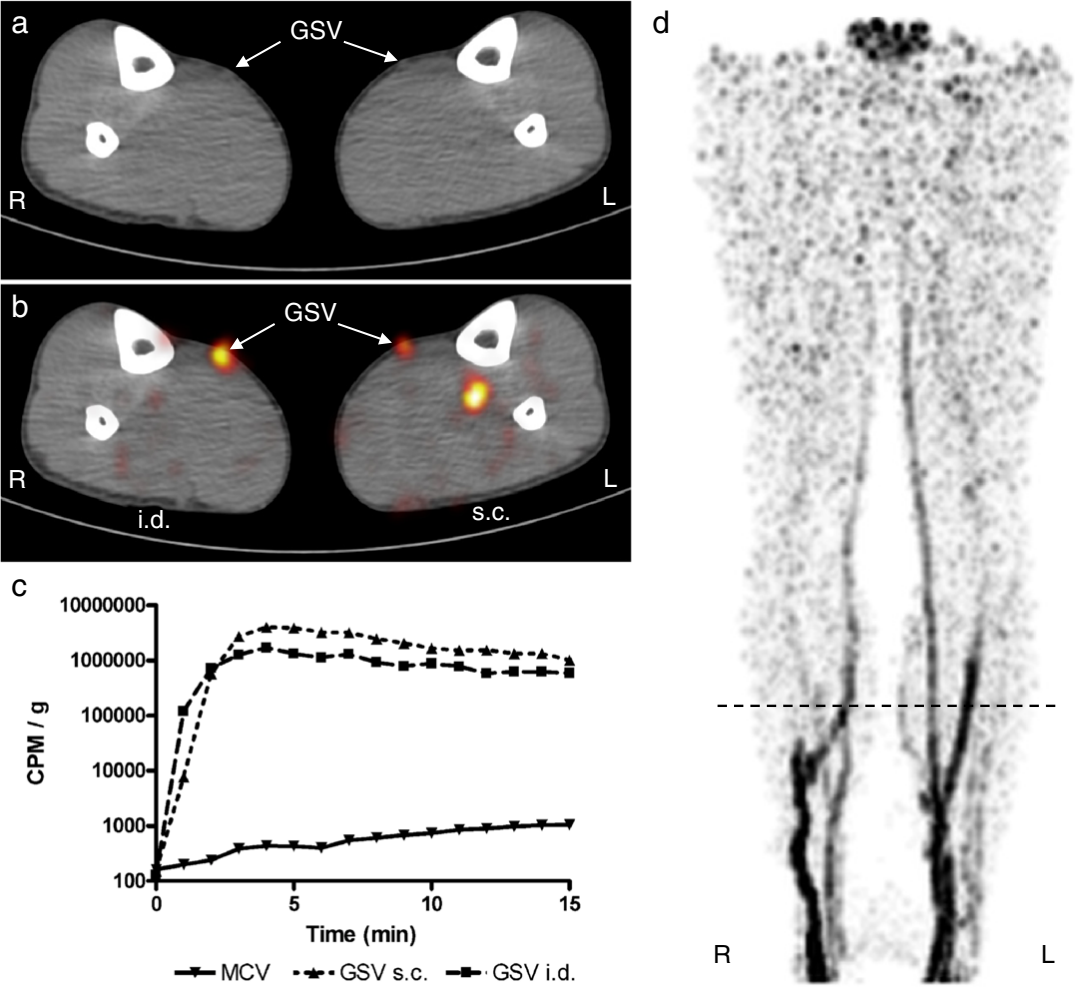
Results for subject 4. For figure details, see legend of Figure 1. A 33-year-old male. ^18^F-FDG activity is visualized in the great saphenous veins bilaterally, but on the left (s.c. depot), also in a deep vein.
